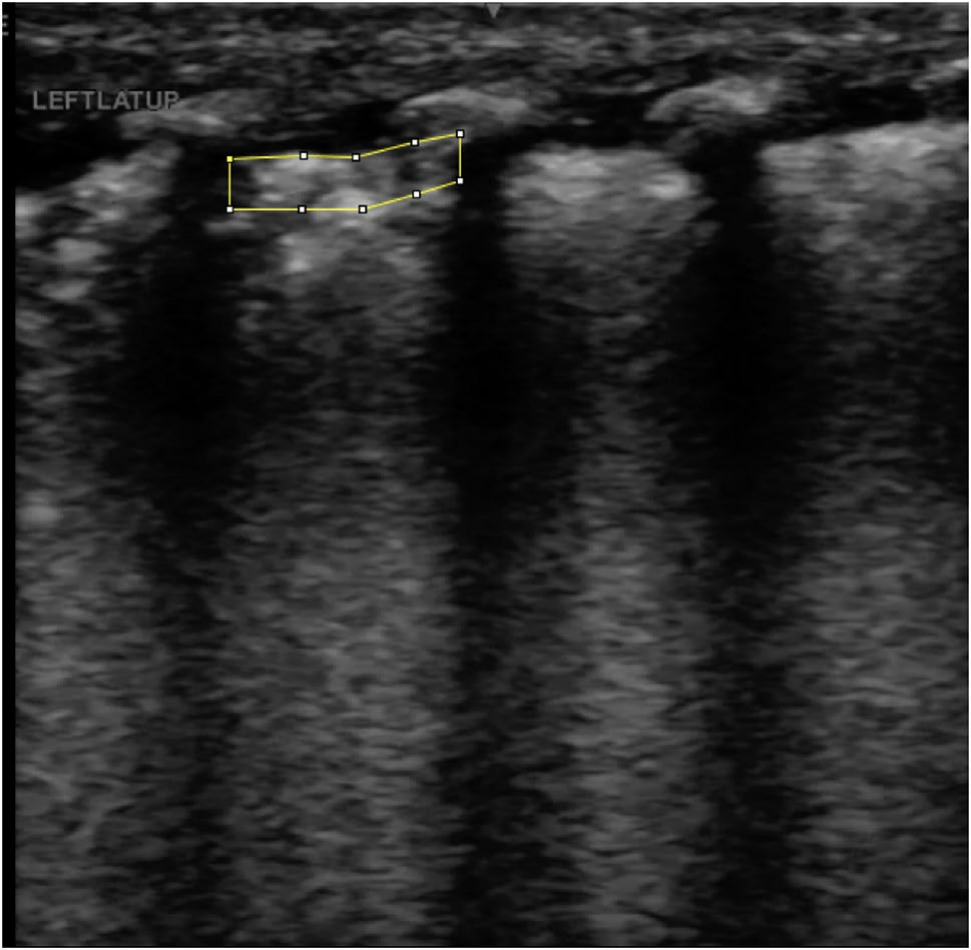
Manual selection of pleural region of interest on lung ultrasound.
